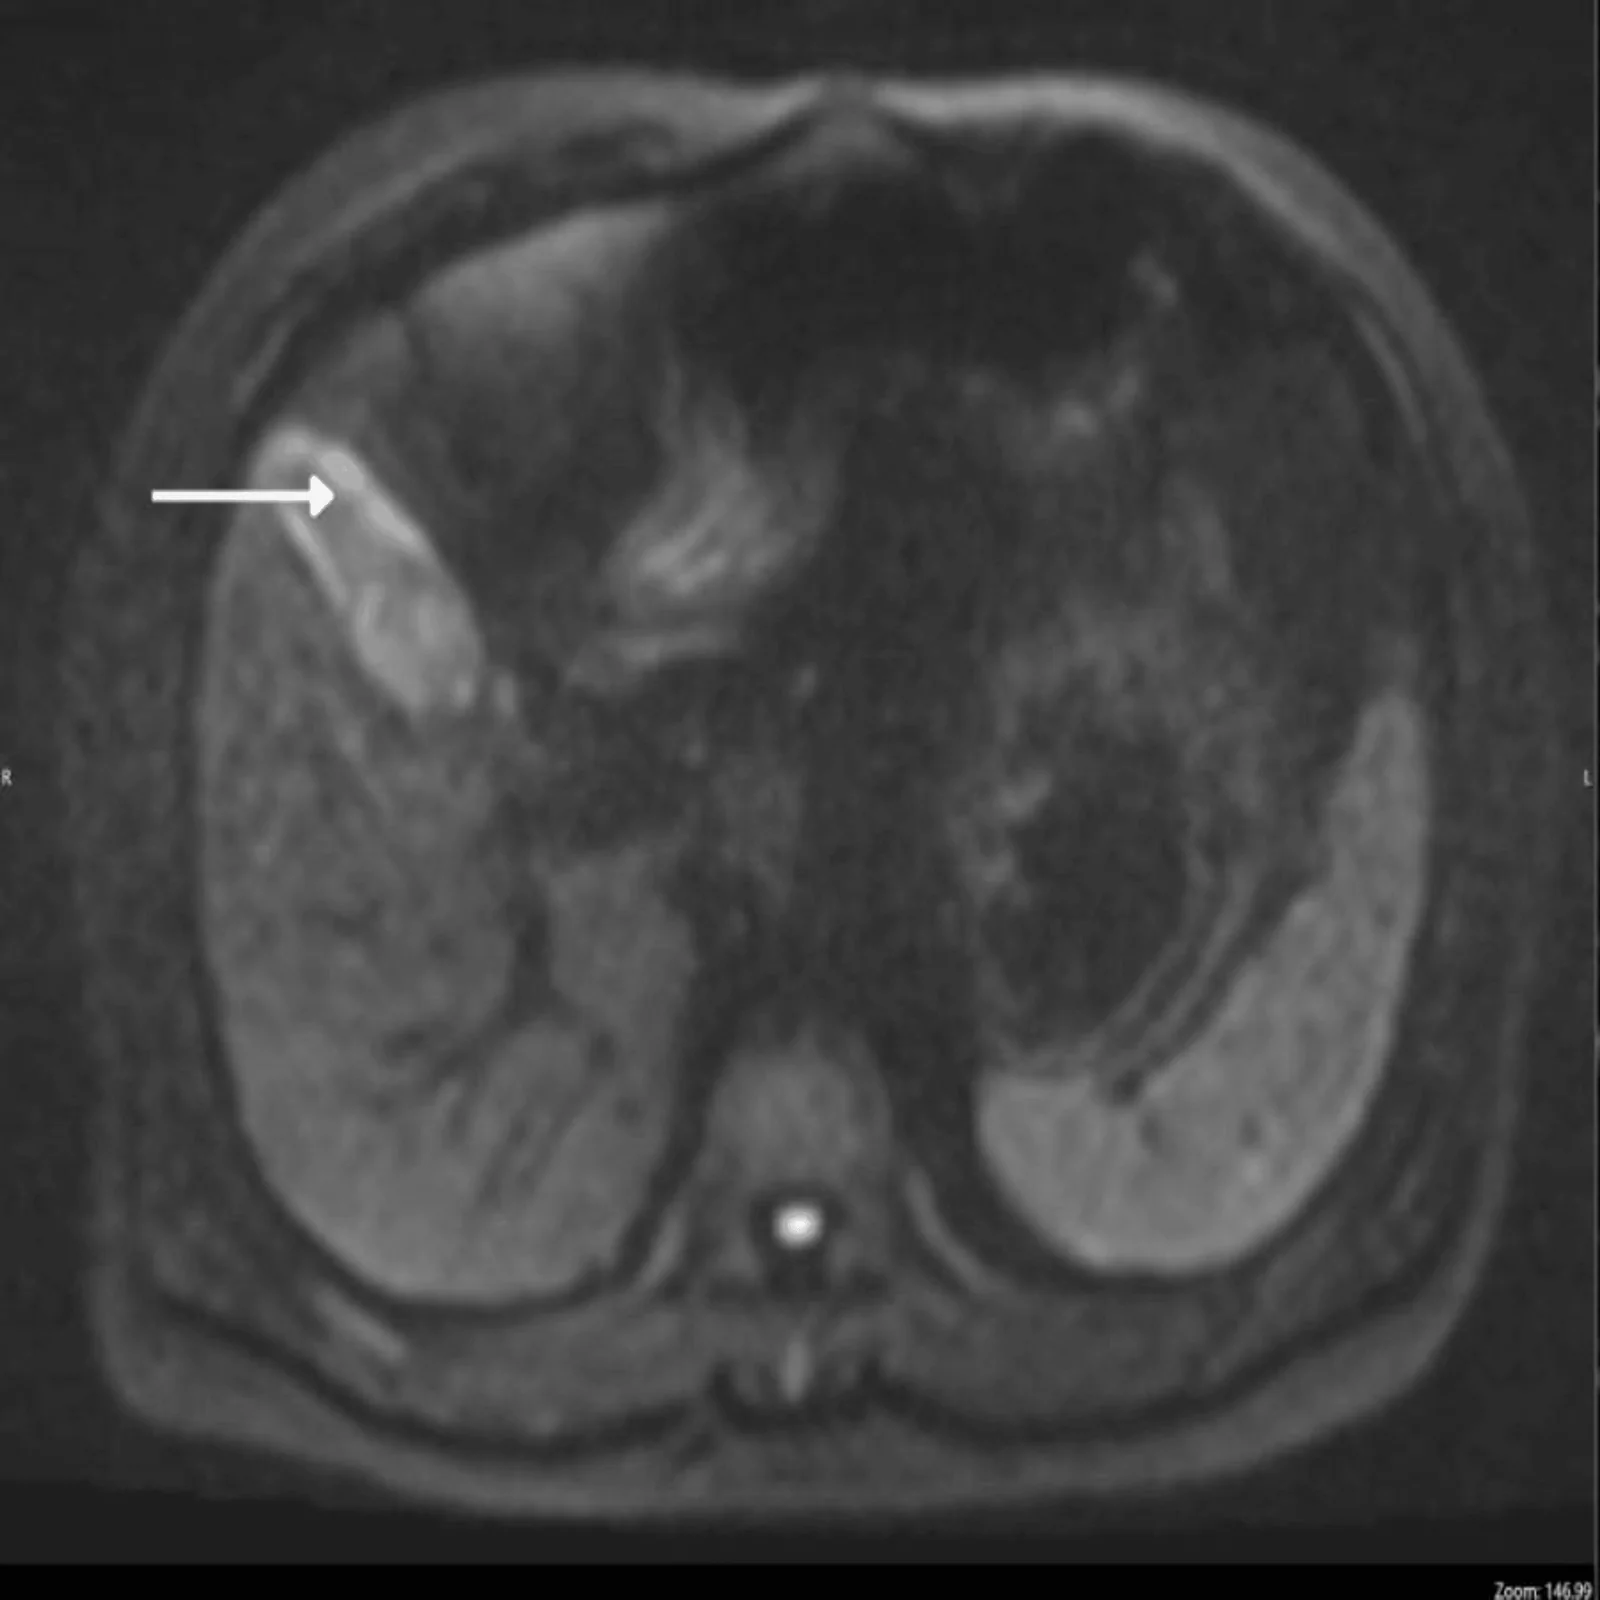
Axial diffusion-weighted imaging (DWI) image showing focal hyperintense wall thickening involving the fundus and distal body of the gallbladder, measuring up to approximately 6 mm The arrowhead points toward the focal wall thickening.
